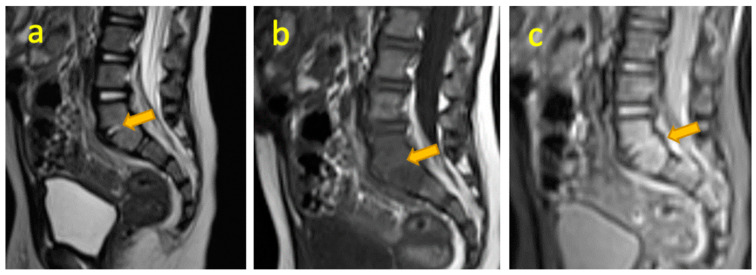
(a–c) A 13-month-old boy presented with a painful limp for 3 weeks and had raised inflammatory markers on a blood test. Sagittal STIR, T1-weighted, and T1W post-contrast fat-saturated images demonstrate acute L5-S1 intervertebral discitis.
